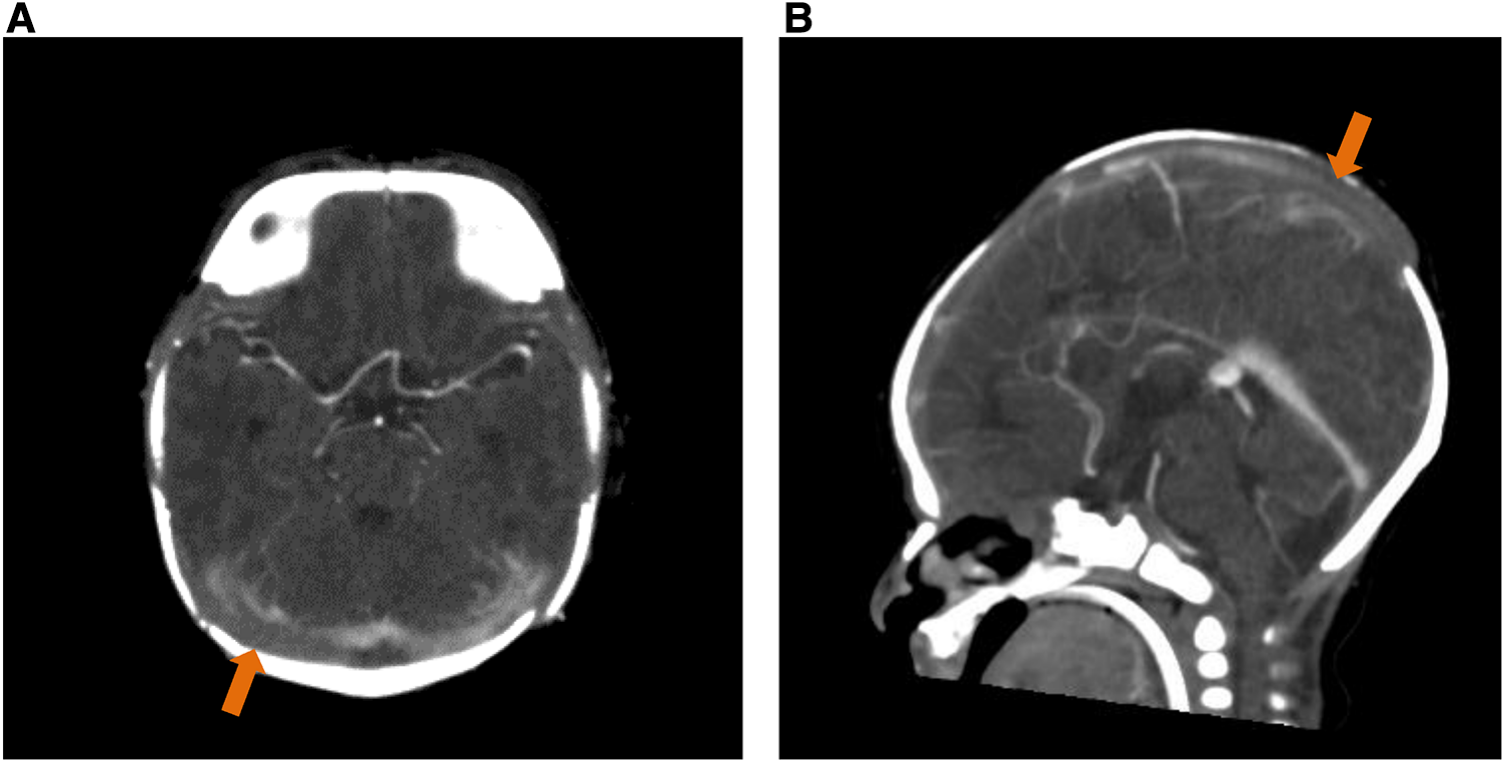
Contrasted brain CT of the patient. (A) Transverse sinus thrombosis. (B) Superior sagittal sinus thrombosis.
